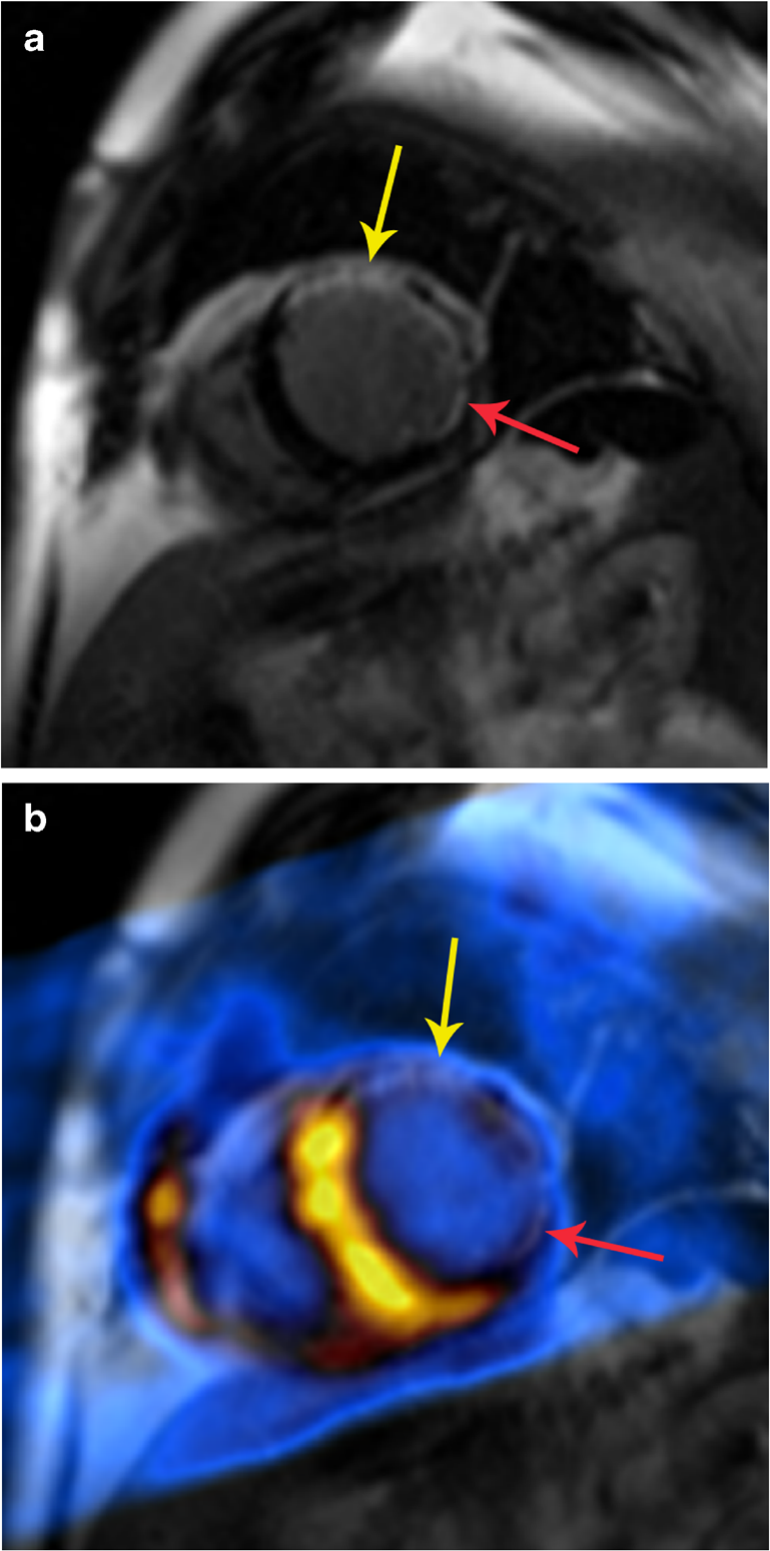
Myocardial infarction. a Short-axis delayed enhancement MRI in a patient with myocardial infarction shows a large transmural scar of the anterior wall (yellow arrow) and partial thickness scarring of the inferolateral segment (red arrow). Viability of this segment is borderline. b Fused PET/MR image shows no uptake in the anterior as well as inferolateral segments, indicating that these are not viable. Thus, the combination of these imaging modalities improves the diagnostic confidence
